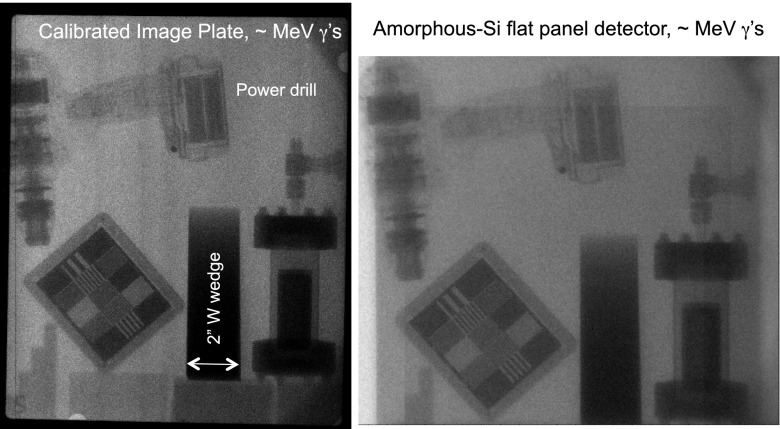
Point projection radiographs of thick objects recorded on collocated image plates (left) and on an a-Si gamma-ray detector (right).
